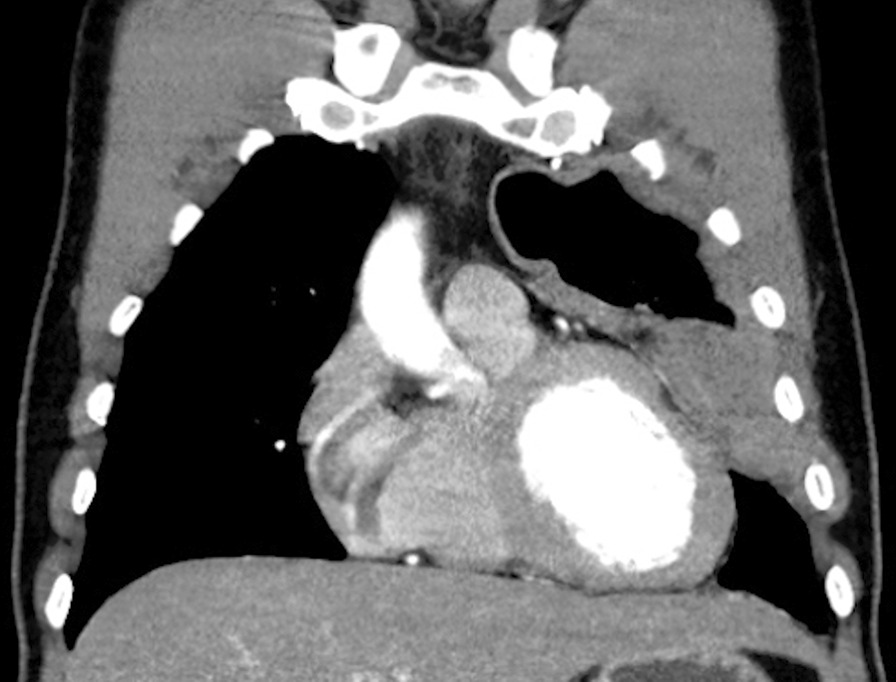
The computed tomography imaging scan shows a tumor mass adjacent to the lateral wall of the left ventricle. This image demonstrates proximity of the tumor mass to the affected myocardium
